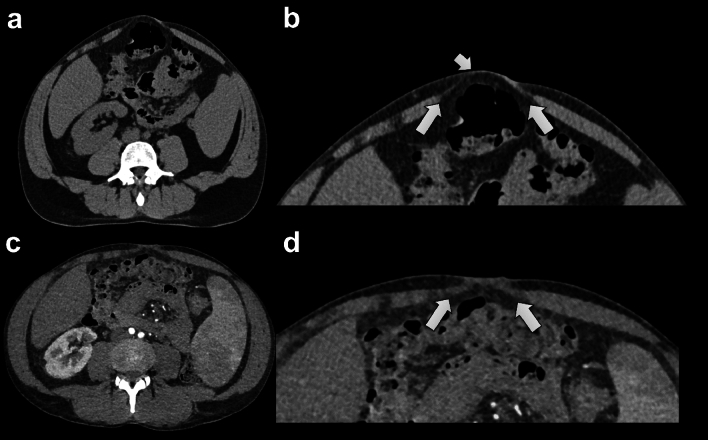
40-year-old male patient with symptomatic diastasis recti. Valsalva CT (A, B) showing widening of the linea alba above the umbilicus (long arrows in B) and bulging (short arrow in B). The inter-rectus distance was 64 mm. A non-Valsalva CT (C, D) done two months earlier for other reasons (to plan for endovascular aortic valve repair) does not depict the bulge and the inter-rectus distance appears smaller at 36 mm (long arrows in D)
